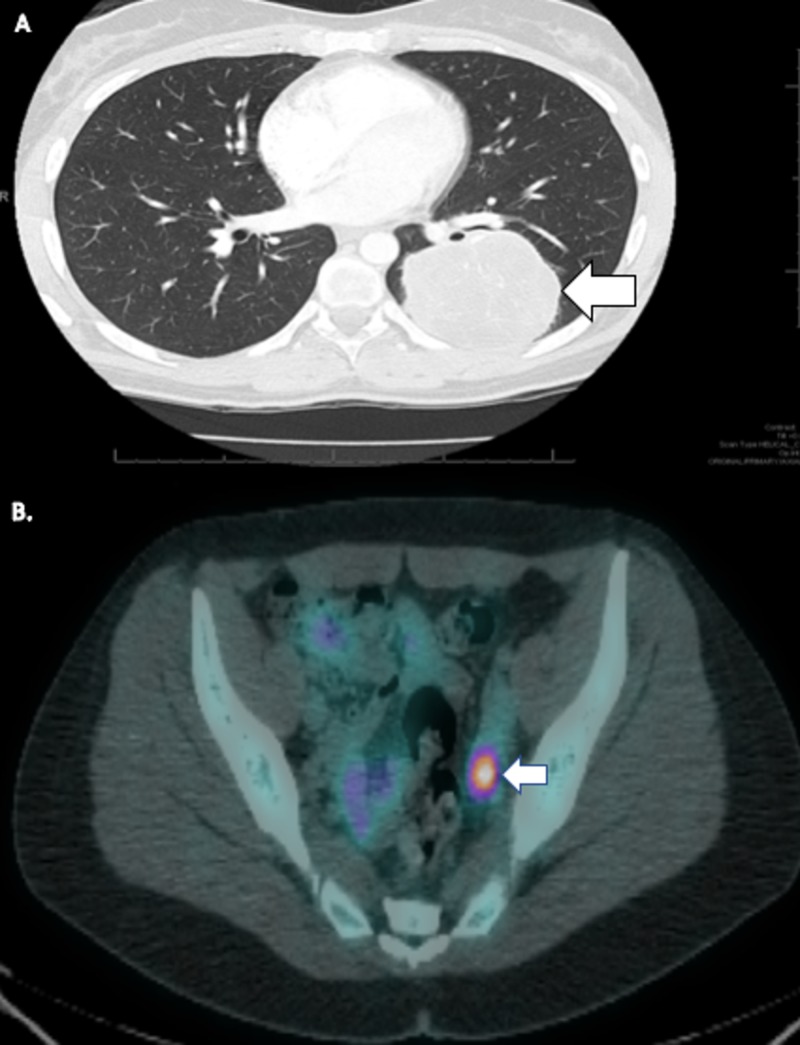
Case 2: baseline imaging (A) Computed tomography of the chest with a 7.7-cm left lower lobe mass. (B) Staging positron emission tomography/computed tomography scan showed a mildly increased uptake in the left adnexal area.
